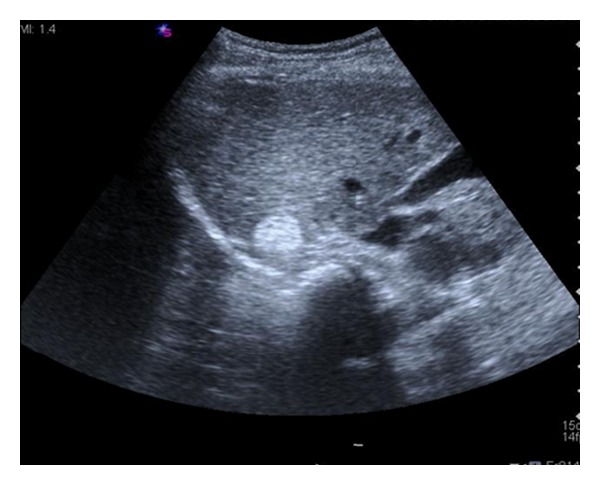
Ultrasonographic findings of the liver mass. Ultrasonogram demonstrates a 2.5 cm sized round, well-marginating hyperechoic mass.
